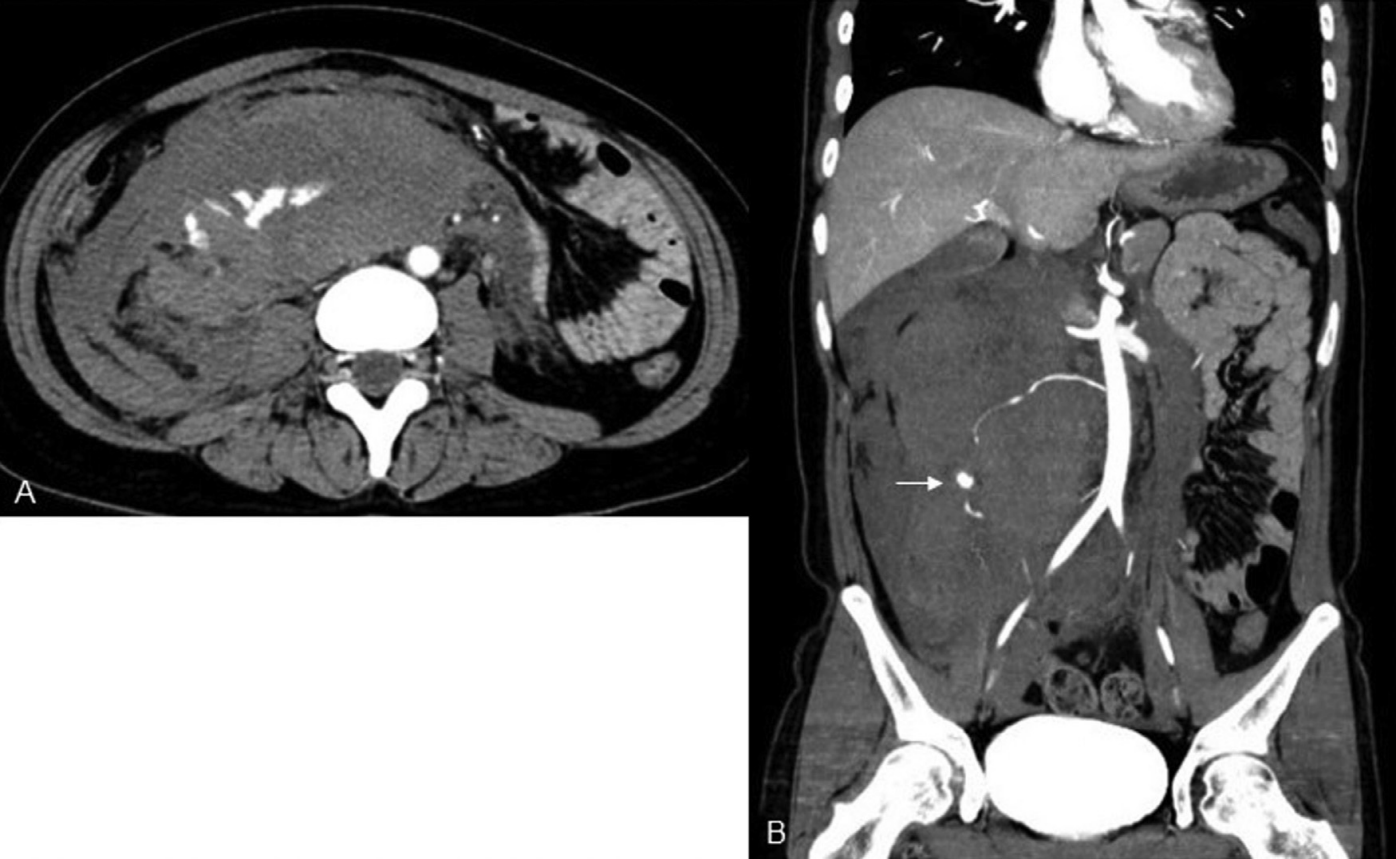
Preoperative computed tomography shows a large retroperitoneal mass with extravasation of contrast extending from the inferior pole of the right kidney to the right pelvic regions (A) and the right ovarian artery with an aneurysm (white arrow) at its middle portion in the center of the mass (B).
